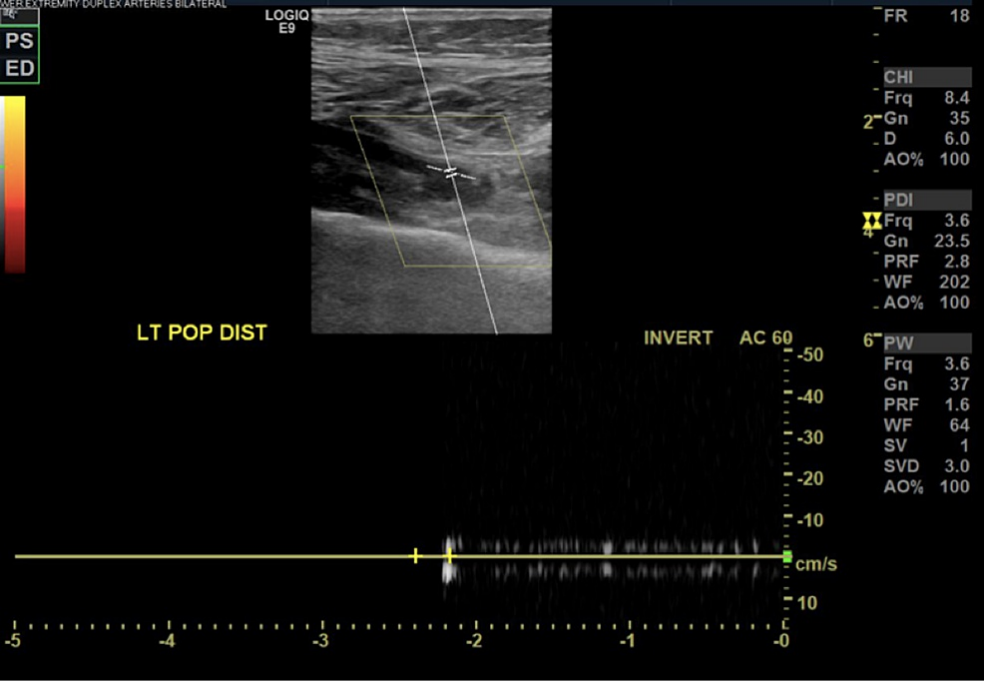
Lower extremity arterial Doppler. No flow in the left distal popliteal artery.
